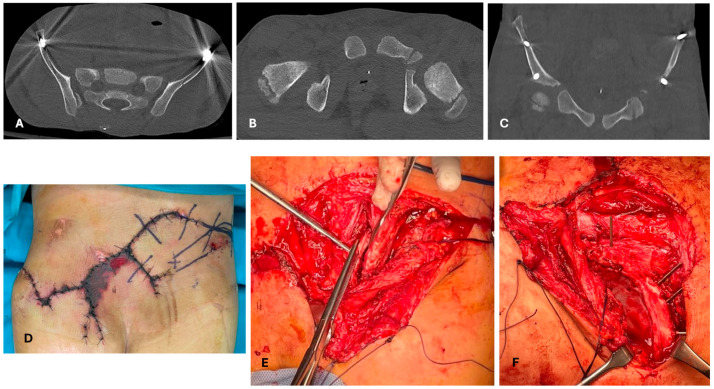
The same patient as Figure 1. CT check for planning, with ExFix in situ. (A–C) Triplanar reconstruction. (D) The image shows the situation at 5 days post-trauma and the time of definitive treatment. (E) Intraoperative findings: the Risser is completely detached from the iliac wing, starting from the posterior-superior iliac spine to the anterior third of the iliac wing. (F) Intraoperative finding after reduction in the iliac wing and Risser; fixation is performed with K-wires [25].
